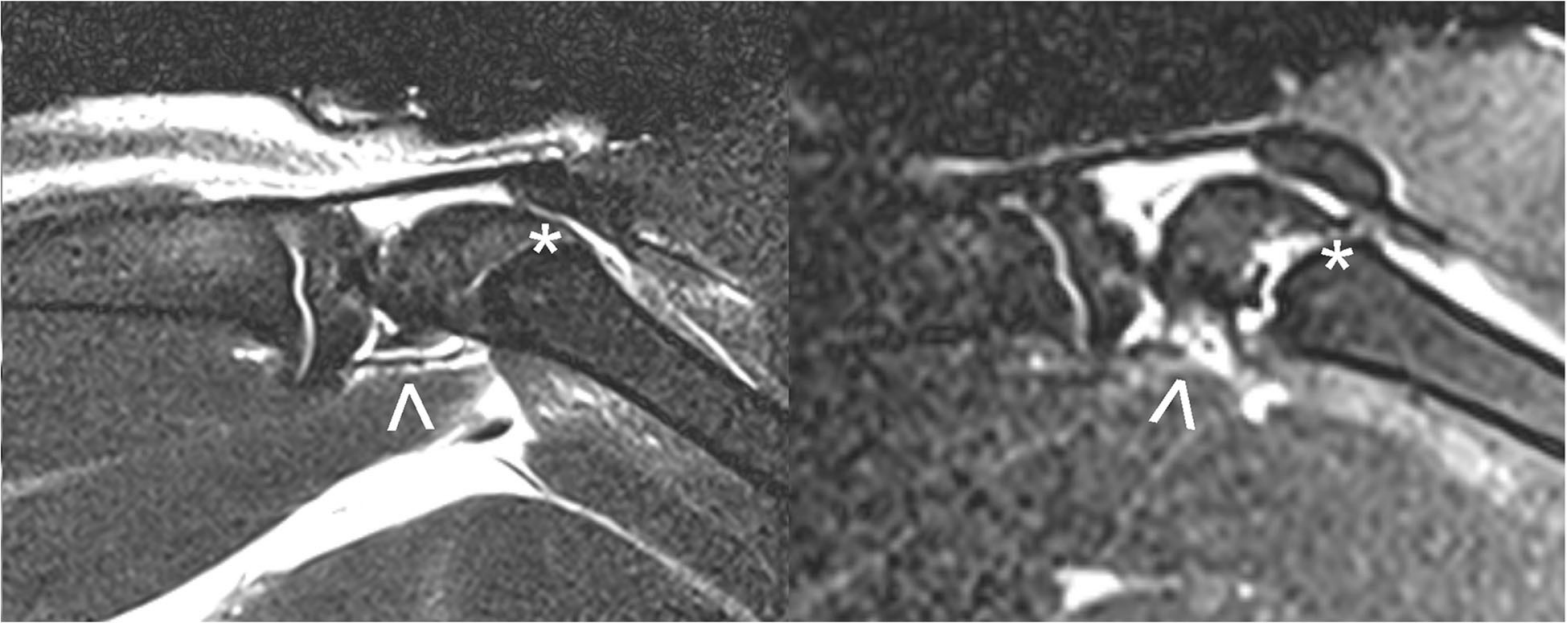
MRI scans of the knee joint. MRI (proton density and T2-weighted turbo spin-echo sequence) scan of the knee joint before (left) and after (right) passive hyperextension of − 45° shows posterior capsular lesion (marked with an arrow) and a posterior widening of the femoral growth plate (anterior part of the growth plate is marked with an asterisk) after the maneuver
